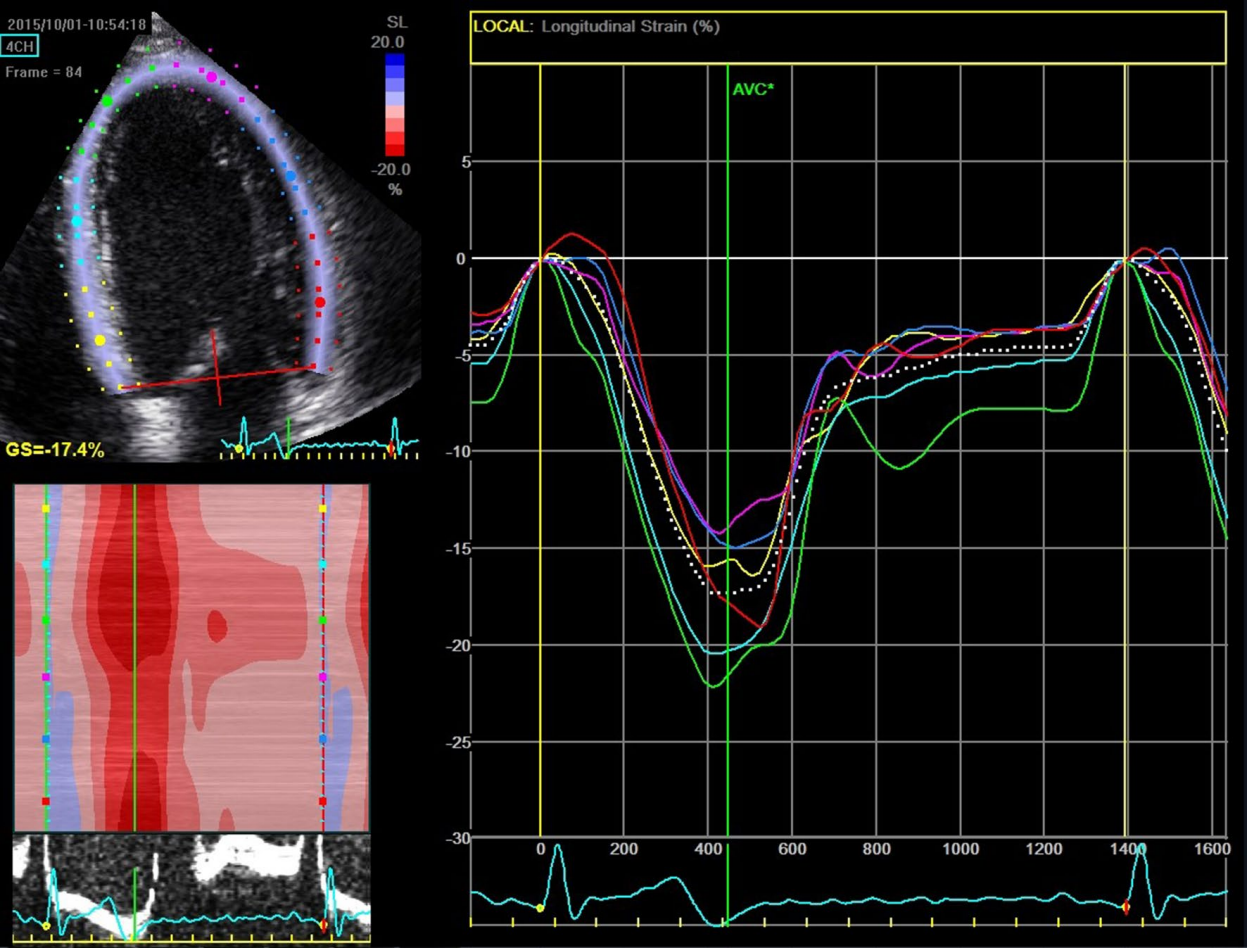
Longitudinal strain curves from apical four-chamber view in a 53-year-old genotype-positive (MYH7 mutation) relative with normal EF (63%). Average strain from four-chamber view was − 17% (dotted line) and GLS was − 18%, indicating reduced longitudinal function
